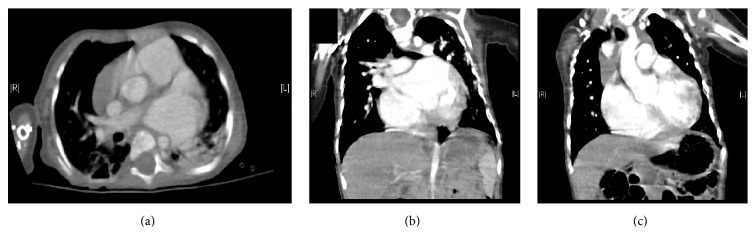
CT scan taken at 7 months: (a) enlarged left atrium and dilated pulmonary trunk. (b) Atrial septal defect. (c) Dilated aortic root and patent ductus arteriosus.
